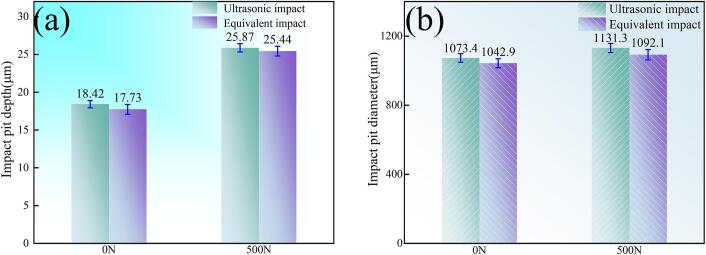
Morphological results of ultrasonic impact and equivalent impact test on 45# steel. (a) Impact pit depth. (b) Impact pit diameter.
